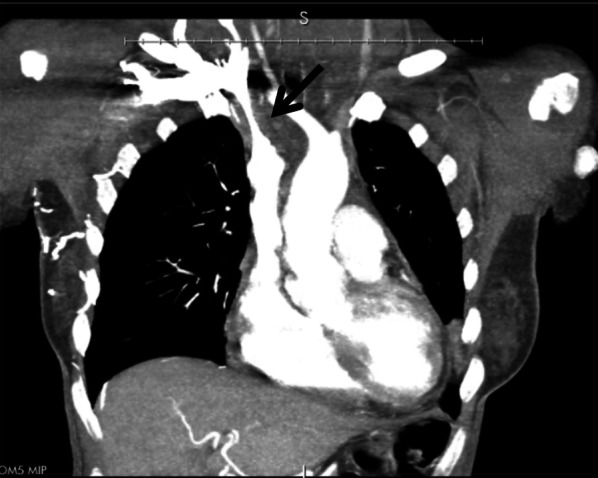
A computed tomography scan of the chest identified significant mediastinal lymphadenopathy (arrow) that compressed the airway and the superior vena cava
